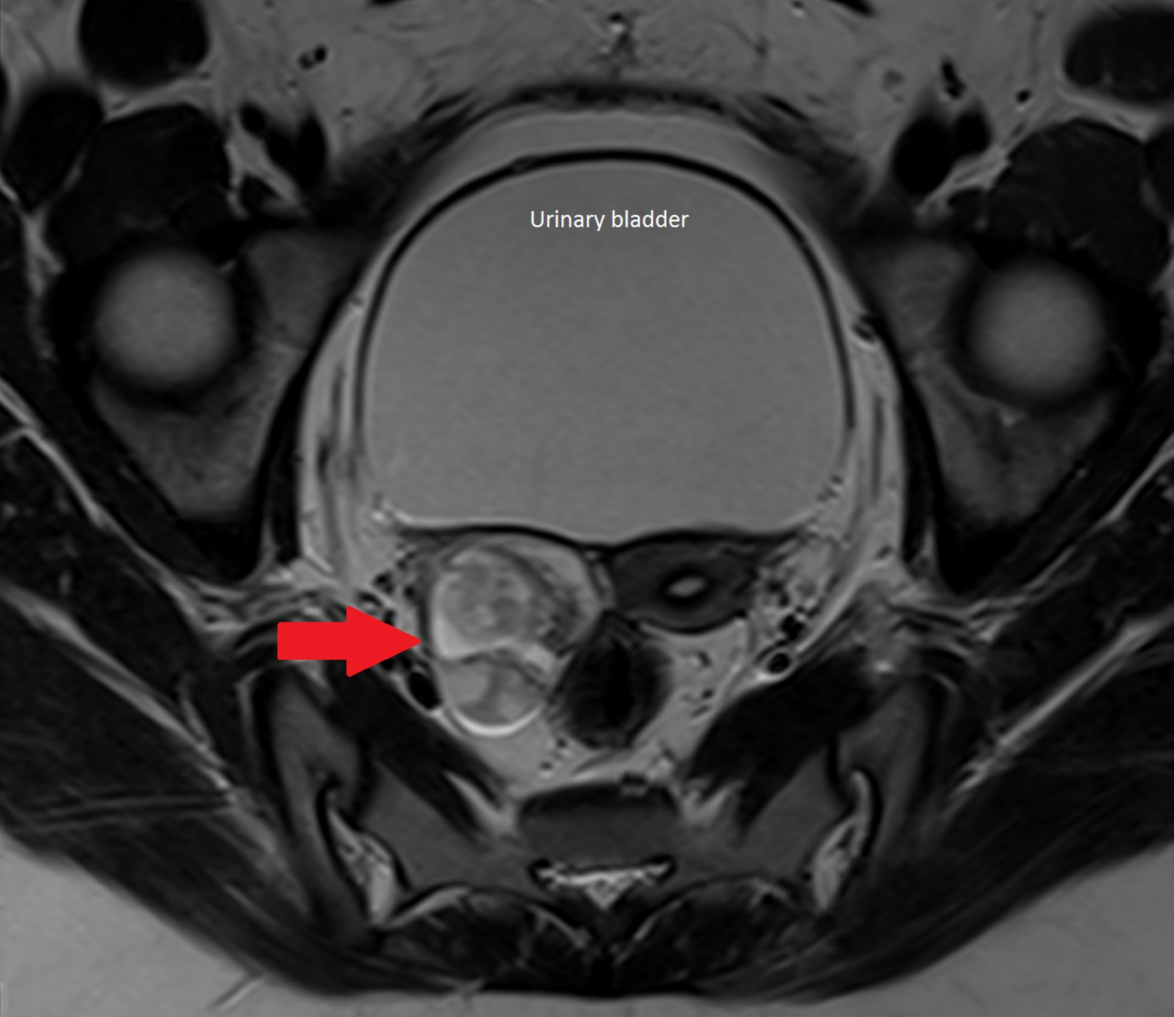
Pelvic MRI Red arrow showing a heterogeneous, complex, mixed solid, and cystic lesion in the right ovary.
